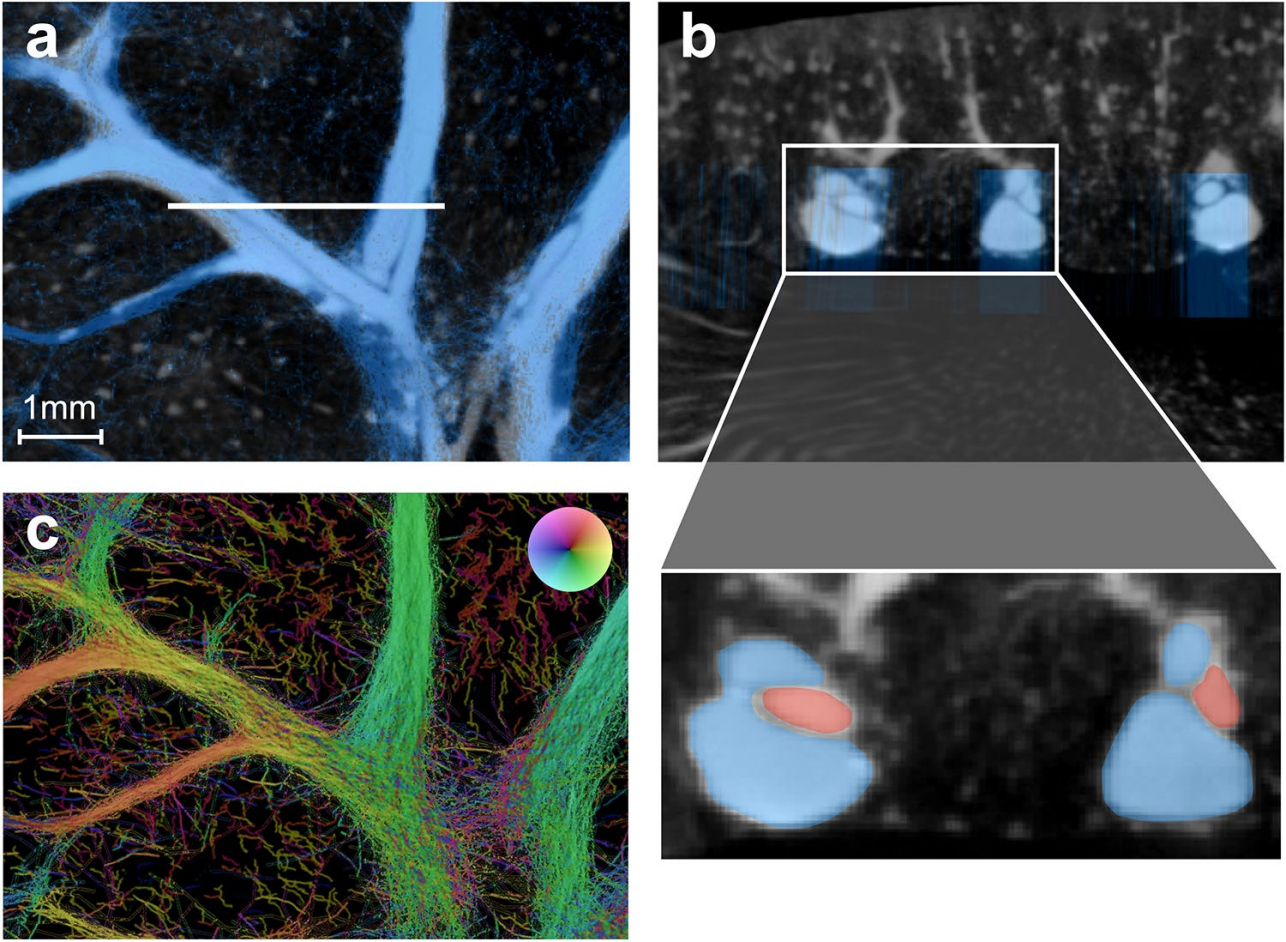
Veins wrapped around their paired artery. (a) Section of a coronal image slice of the *μ*CT with overlap of super-resolution ultrasound scan 2 (blue). (b) Section of a sagittal image slice of the *μ*CT with overlap of super-resolution ultrasound scan 2 (blue). The up-scaled content shows how the large segmental veins (blue) wrap around their paired segmental artery (red). (c) Unfiltered microbubble track map of the same slice as (a) (for visualization, the color transparency in the microbubble track map is scaled by the intensity map). The arteries are not readily apparent in the microbubble track maps without filtering and separating the microbubbles with artery and vein flow direction, as shown in Fig. 3b-2.
